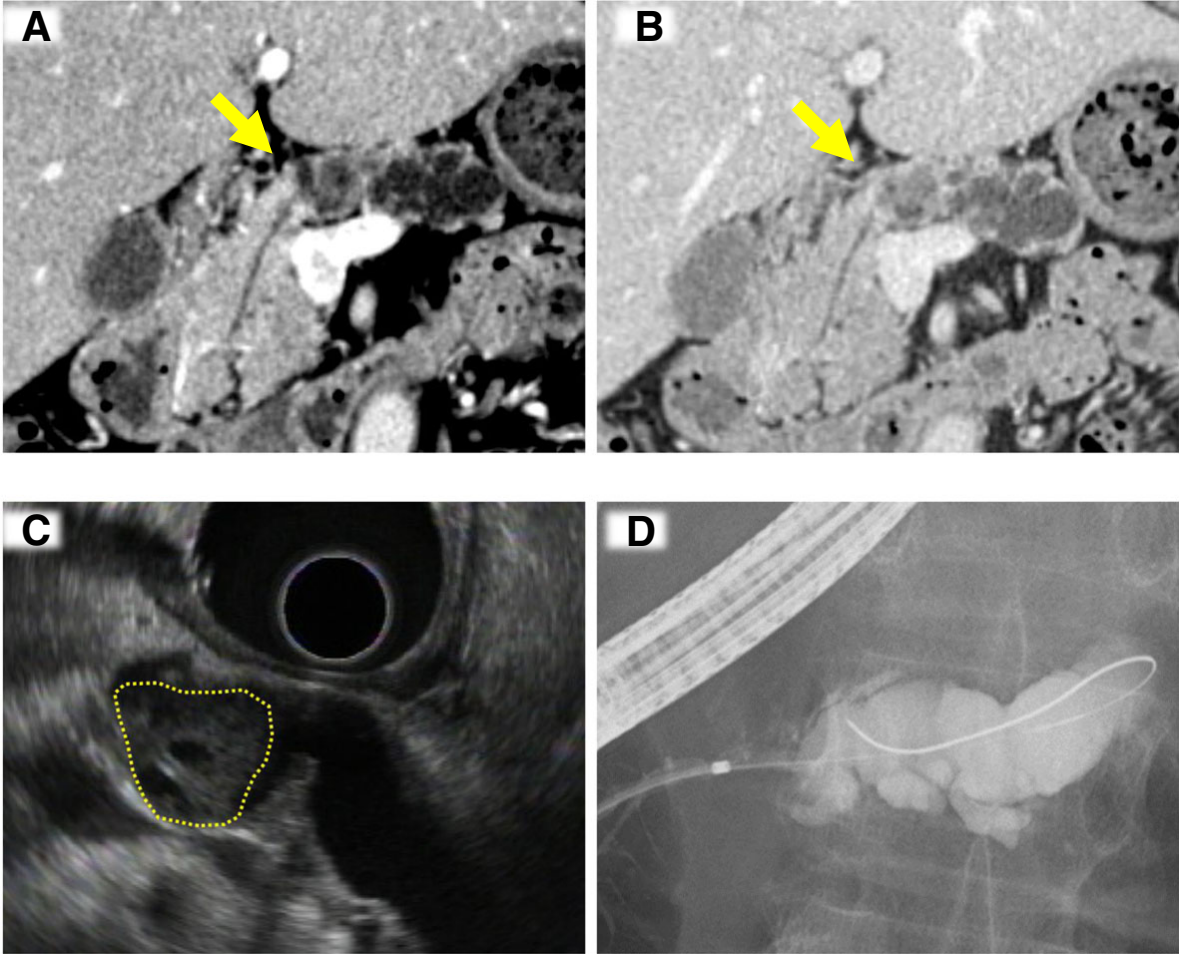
Radiologic findings. a, b Dynamic computed tomography. A solid mass in the pancreatic body (indicated by an arrow) is enhanced at the arterial phase (a), and the enhancement is prolonged at the delayed phase (b). c Endoscopic ultrasonography. A tumor developed in the main pancreatic duct (a dotted circle). d Endoscopic retrograde cholangiopancreatography. The main pancreatic duct of the distal portion is markedly dilated because the solid tumor obstructs fluid passage
